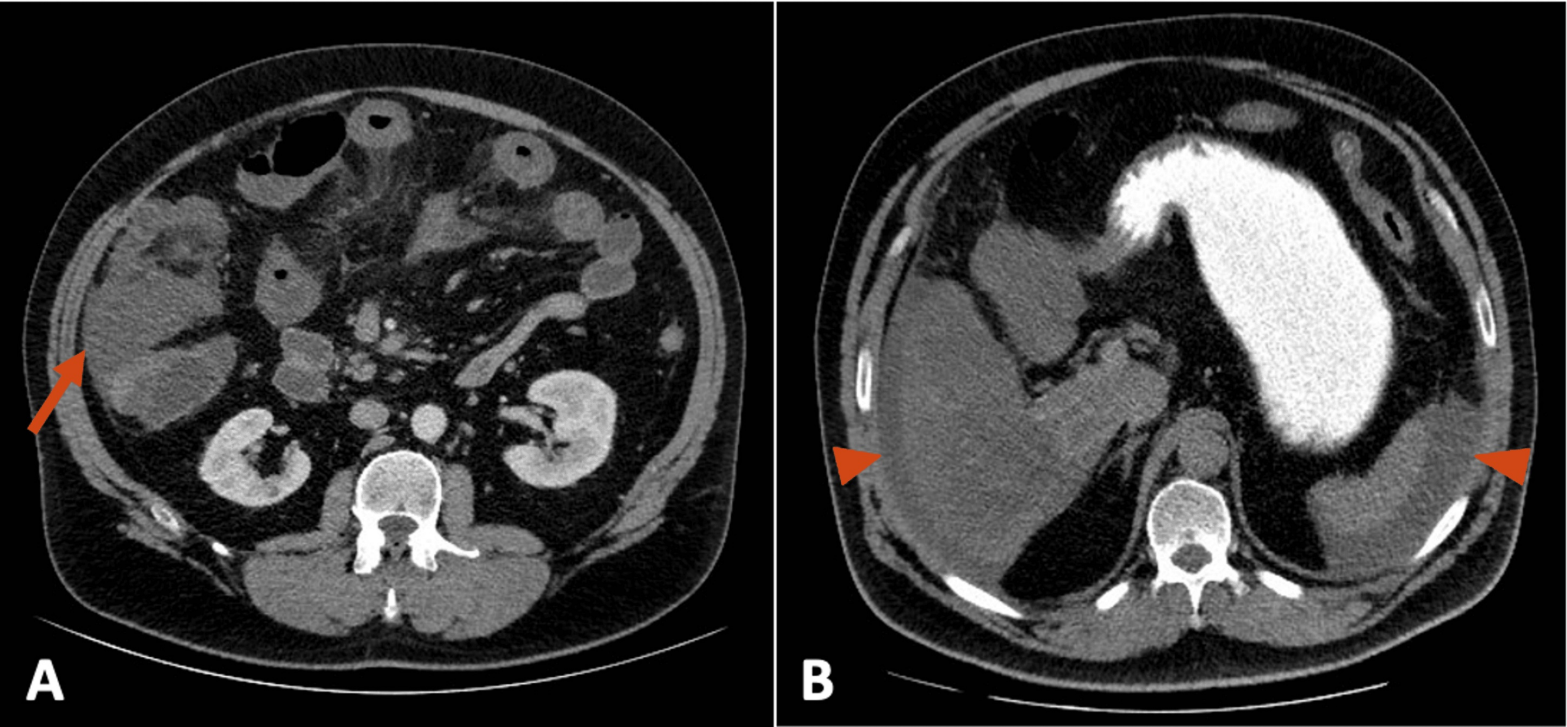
Preoperative abdominal CT scan. (A) Contrast-enhanced image showing edema and thickening of the cecal wall and ileocolic valve (arrow), with associated ileal loop distension. (B) Oral-contrast image demonstrating free peritoneal fluid (arrowhead).
